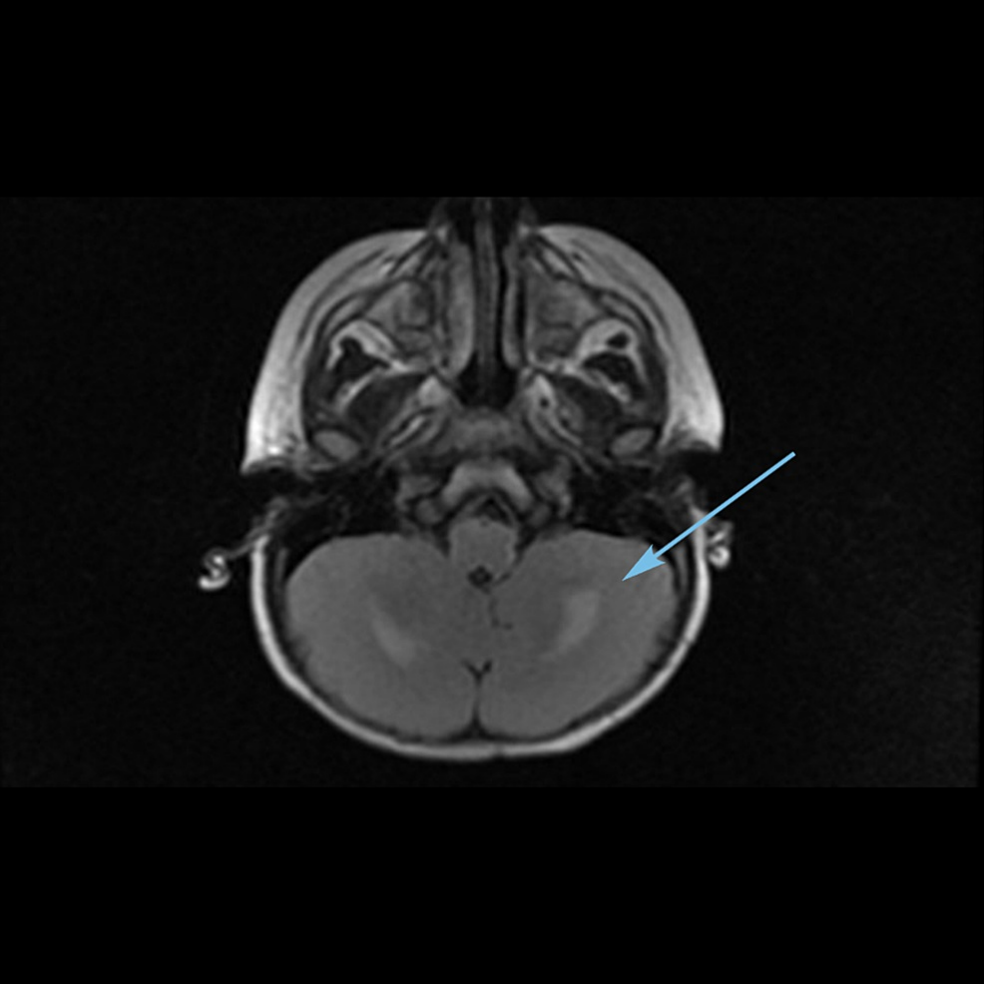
MRI brain axial T2-weighted image showing hyperintensities in the dentate nuclei (arrow).
